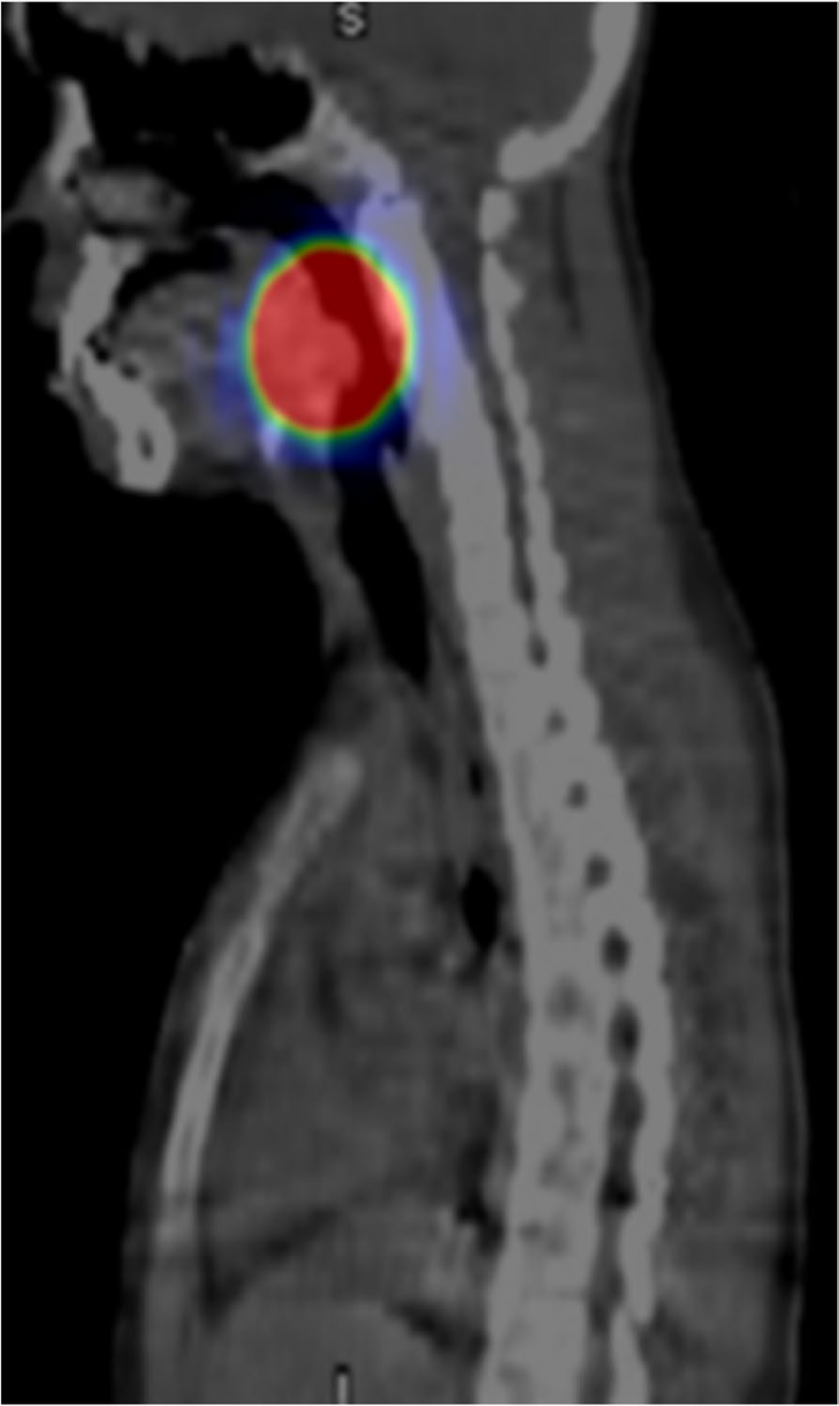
Postoperative ^123−^I scintigraphy shows uptake in the sublingual lesion. This result proved that the sublingual mass was an ectopic thyroid
